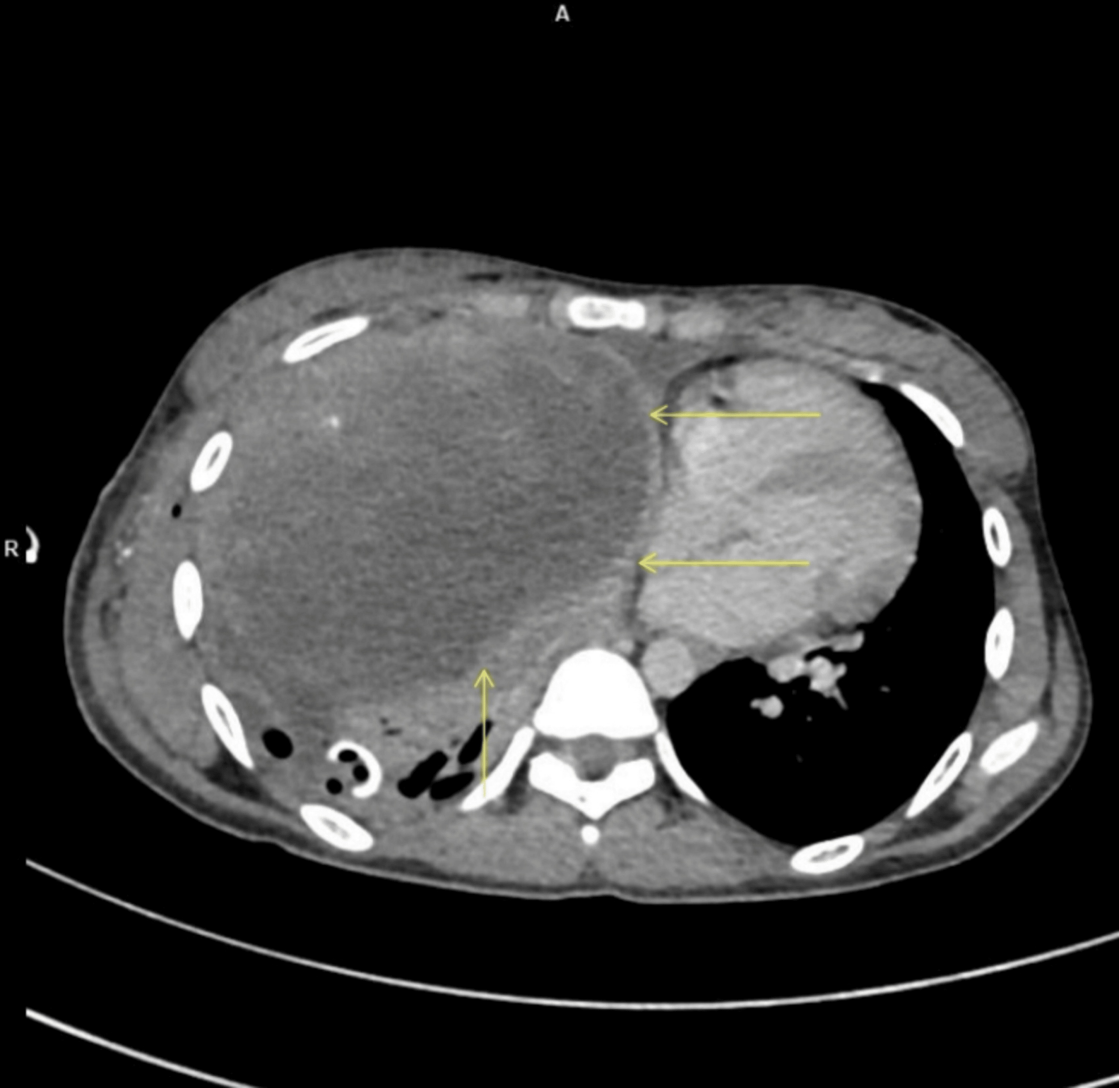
Contrast-enhanced CT thorax (axial view) demonstrating a peripherally enhancing lesion in the right hemithorax (arrows).
